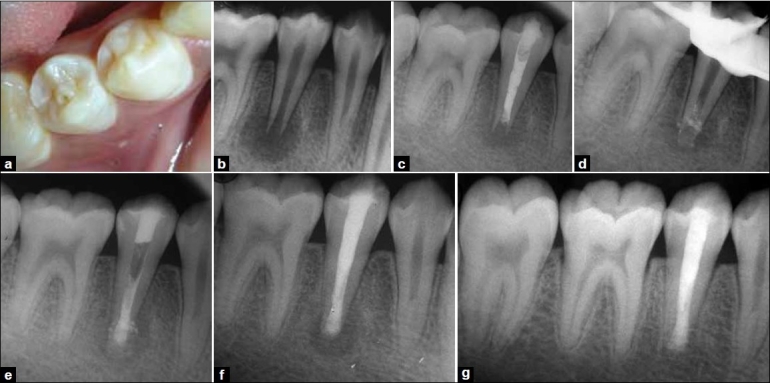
(a) Right mandibular second premolar showing the presence of dense evaginatus, (b) Preoperative radiograph of right mandibular second premolar showing deep pit communicating with pulp space along with blunderbuss canal and large periapical radiolucency, (c) 15 days recall radiograph after the placement of calcium hydroxide and iodoform paste, (d) Apical matrix of decalcified freeze dried bone allograft, (e) Apical plug of MTA created over the apical matrix, (f) 3 months followup radiograph showing reduction in the size of the periapical lesion, (g) 2 years follow-up radiograph representing complete resolution of the periapical lesion
